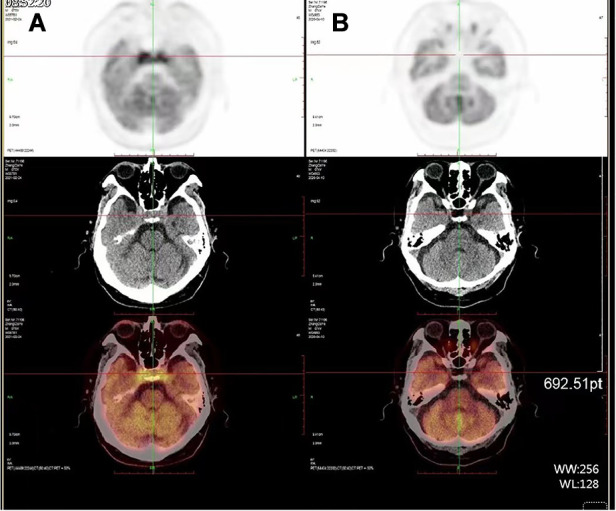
PET-CT scan (A) compared with the images 10 months ago (B), CT scan shows isointense soft tissue filling the suprasellar region, while FDG accumulation in bilateral cavernous sinuses.
